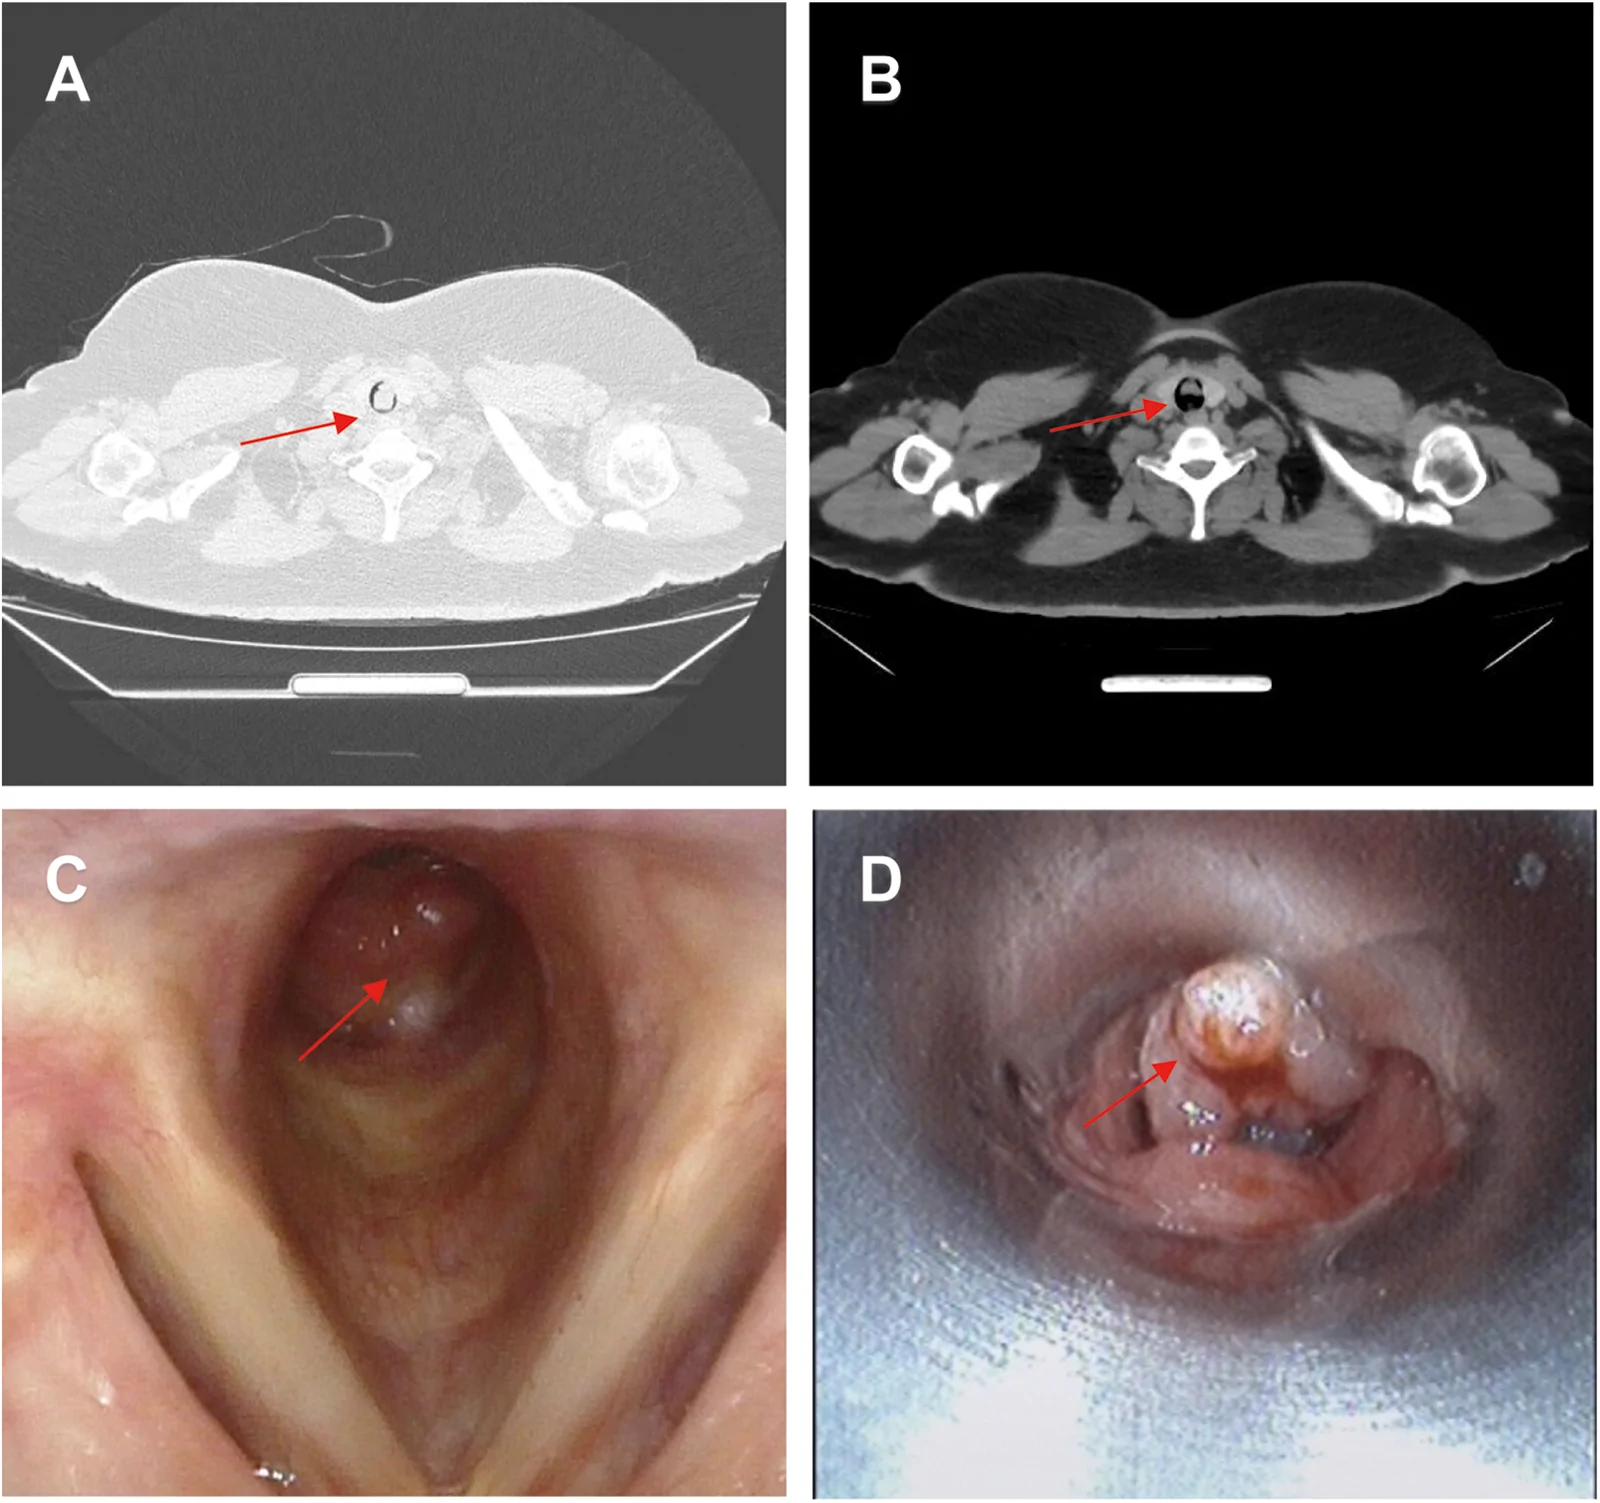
CT and endoscopic findings of the proximal cervical tracheal lesion. (A,B) Axial computed tomography images showing a high-density intraluminal lesion arising from the left anterior wall of the proximal cervical trachea, resulting in marked luminal narrowing. The red arrows indicate the lesion; (C) Flexible laryngoscopic view showing the laryngeal inlet and a polypoid lesion visible distal to the vocal cords, with no definite involvement of the true subglottic mucosa; (D) Rigid bronchoscopic view showing a cauliflower-like polypoid lesion within the proximal cervical tracheal lumen before cryoresection.
